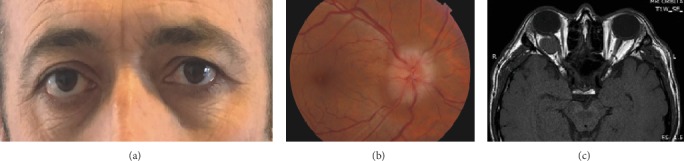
(a) Facial appearance of the patient at presentation. (b) Color fundus photograph of the right eye shows prominent optic disc edema and subtle macular compression. (c) Orbital T1-weighted axial magnetic resonance imaging shows an intraconal tumor isointense to the extraocular muscles producing compression of the globe and indenting the optic nerve.
